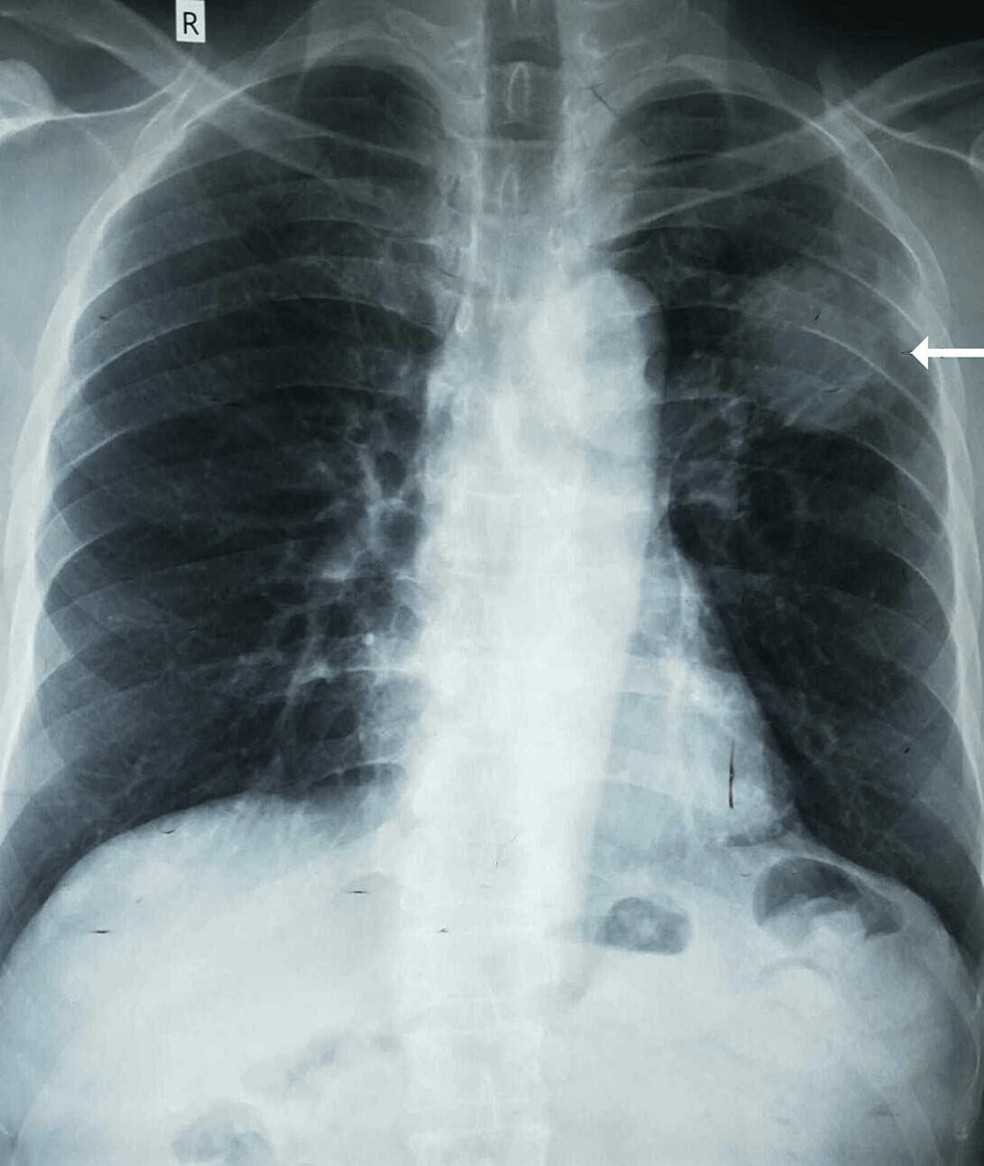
Chest radiographic image showing a mass lesion in the upper lobe of the left lung (white arrow).
